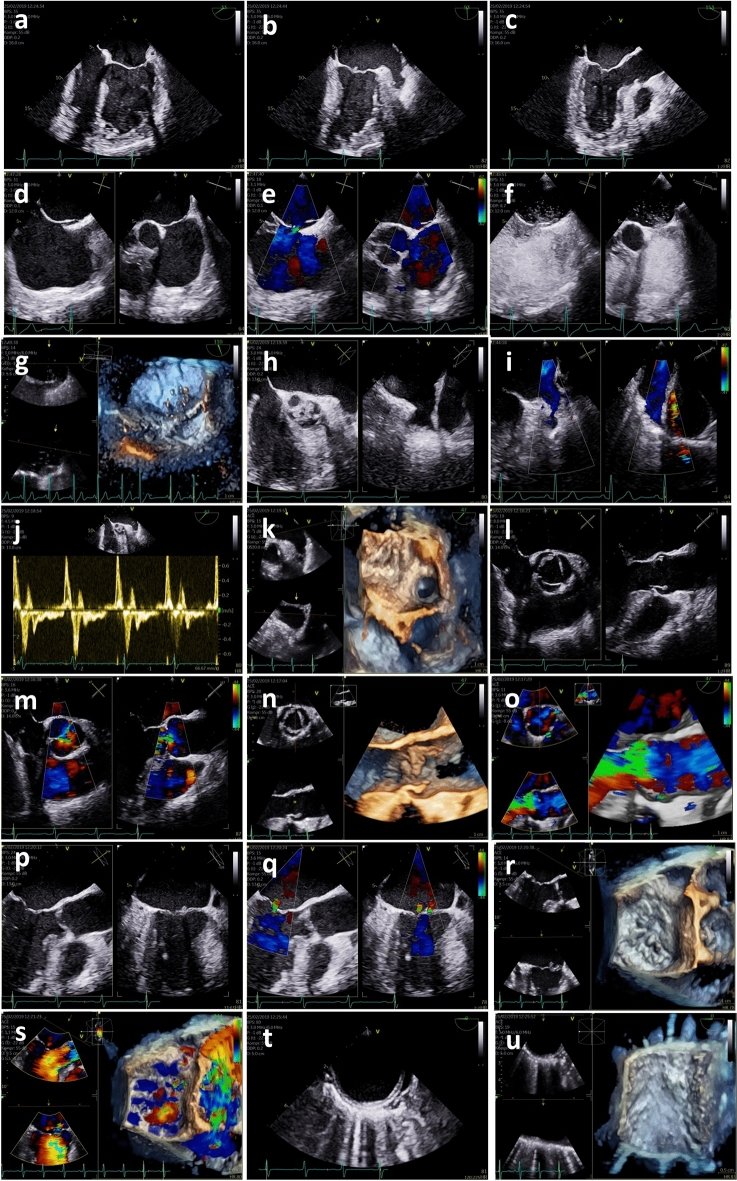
Image acquisition transoesophageal echocardiography protocol in post-stroke patients. Midoesophageal 4-chamber view for assessment of LV function using deformation imaging, if documentation using transthoracic echocardiography (TTE) is not possible (a); midoesophageal 2-chamber view for assessment of LV function using deformation imaging, if TTE documentation is not possible (b); midoesophageal long-axis view for assessment of LV function using deformation imaging, if TTE documentation is not possible (c); conventional 2D-documentation at least in 2 different sectional planes of the interatrial septum (IAS) (d); color-coded 2D-documentation at least in 2 different sectional planes of the IAS to document interatrial communication defects (e); contrast 2D-documentation with agitated saline at least in 2 different sectional planes of the IAS to document patent foramen ovale (PFO). Ideally no or mild sedation of the patient is performed and the Valsalva maneuver practiced with the patient before administration of agitated saline contrast agent. If available, 3D transoesophageal probes should be used and the test repeated several times, if negative. Good documentation is necessary to distinguish inter-atrial from trans-pulmonary shunts (f); contrast 3D-documentation with agitated saline to document PFO, if possible (g); conventional 2D-documentation at least in 2 different sectional planes of the left atrial appendage (LAA) (h); color-coded 2D-documentation at least in 2 different sectional planes of the LAA to exclude or document LAA thrombus formation (i); pulsed wave (pw) Doppler spectrum of the LAA flow velocities (j); 3D-documentation of the LAA, if possible (k); conventional 2D-documentation of the long axis and short axis view of the aortic valve (AV) (l); color-coded 2D-documentation of the long axis and short axis view of the AV (m); 3D-documentation of the AV and the aortic root complex, if possible (n); color-coded 3D-documentation of the AV and the aortic root complex, if relevant AS or AR is present and if possible (o); conventional 2D-documentation of the long axis and short axis view of the mitral valve (MV) (p); color-coded 2D-documentation of the long axis and short axis view of the MV (q); 3D-documentation of the MV, if possible (r); color-coded 3D-documentation of the MV, if relevant MS or MR is present and if possible (s); conventional 2D-documentation of the descending aorta/aortic arch (t); 3D-documentation of the descending aorta/aortic arch, if possible (u). *AR* aortic valve regurgitation, *AS* aortic valve stenosis, *AV* aortic valve, *IAS* interatrial septum, *LAA* left atrial appendage, *LV* left ventricular, *MR* mitral valve regurgitation, *MS* mitral valve stenosis, *MV* mitral valve, *PFO* patent foramen ovale, *pw* pulsed wave, *TTE* transthoracic echocardiography
